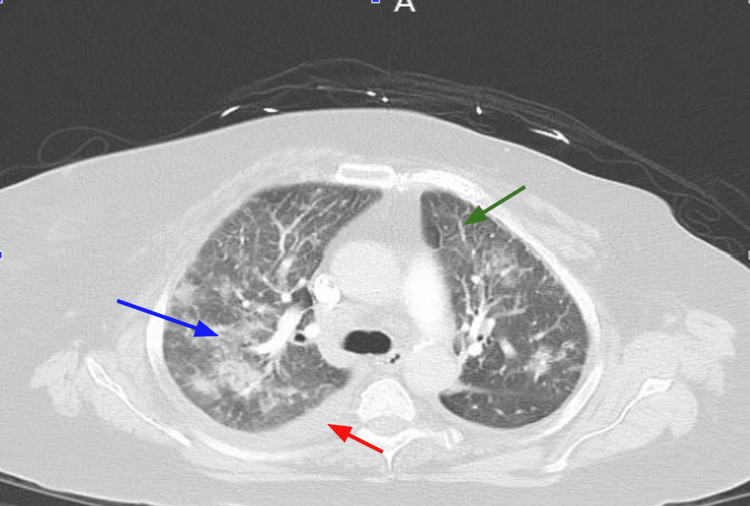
CTA pulmonary showing patchy peribronchovascular opacification (blue arrow), pleural effusion (red arrow), and smooth septal thickening (green arrow).
